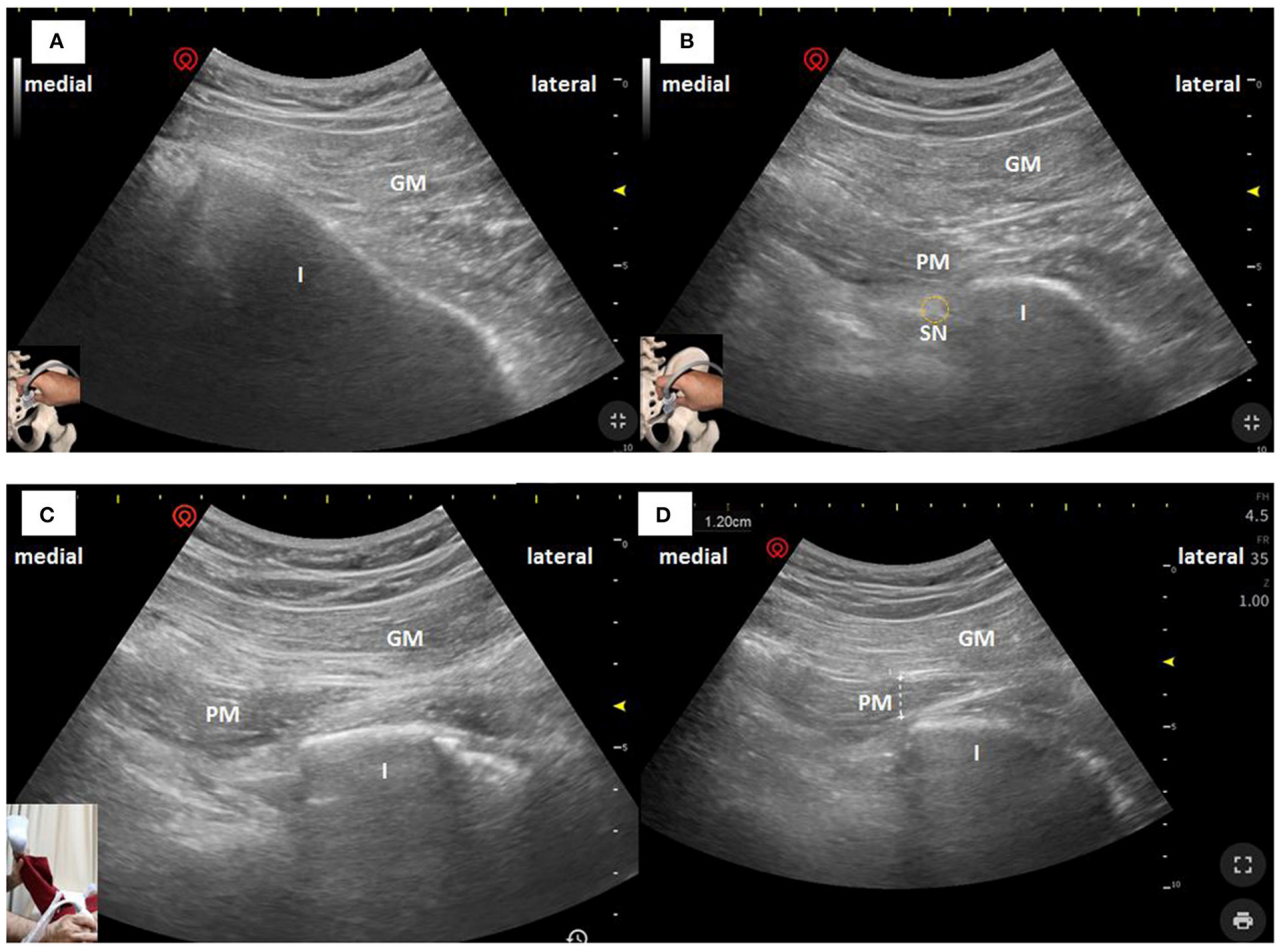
Ultrasonographic images of the PM in the longitudinal plane obtained with a curvilinear transducer at 2.5–5 MHz. The PM can be seen deeper to the GM and passing laterally toward the GT. (A) US image when superficial images above conducted. (B) US image when the clinician moved the probe inferiorly. (C) US image when the patient's leg was maximal abducted. (D) PM thickness was measured in the medial part of the tip of the ischium, which was parallel to the longitudinal plane at the sciatic notch with the patient's leg was abducted 45°. I, Ischium; GM, Gluteus Maximus; PM, Piriformis Muscle; SN, Sciatic Nerve.
